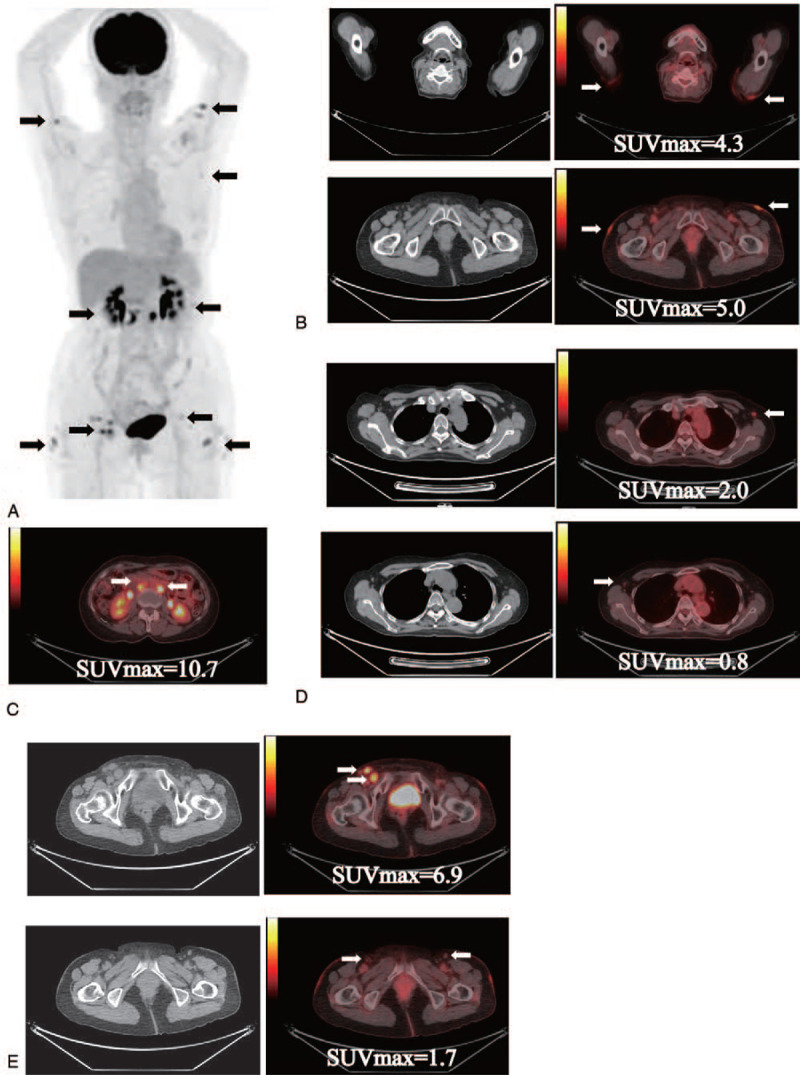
PET-CT imaging of an ovarian cancer patient treated with Trio vaccine. A 65-year-old female with an ovarian cancer underwent an extensive hysterectomy and subsequent chemotherapy. This was followed by continuous administration of the WT1 Trio vaccine therapy for the prevention of relapse. PET-CT imaging was performed as a routine check at the 17th months of the WT1 Trio vaccine therapy. (A) Axial image and coronal images of (B) skin of upper arm and femur, (C) para-aortic lymph nodes, (D) axillary lymph nodes and (E) inguinal lymph nodes. Arrows represent the regions of increased FDG uptakes.
